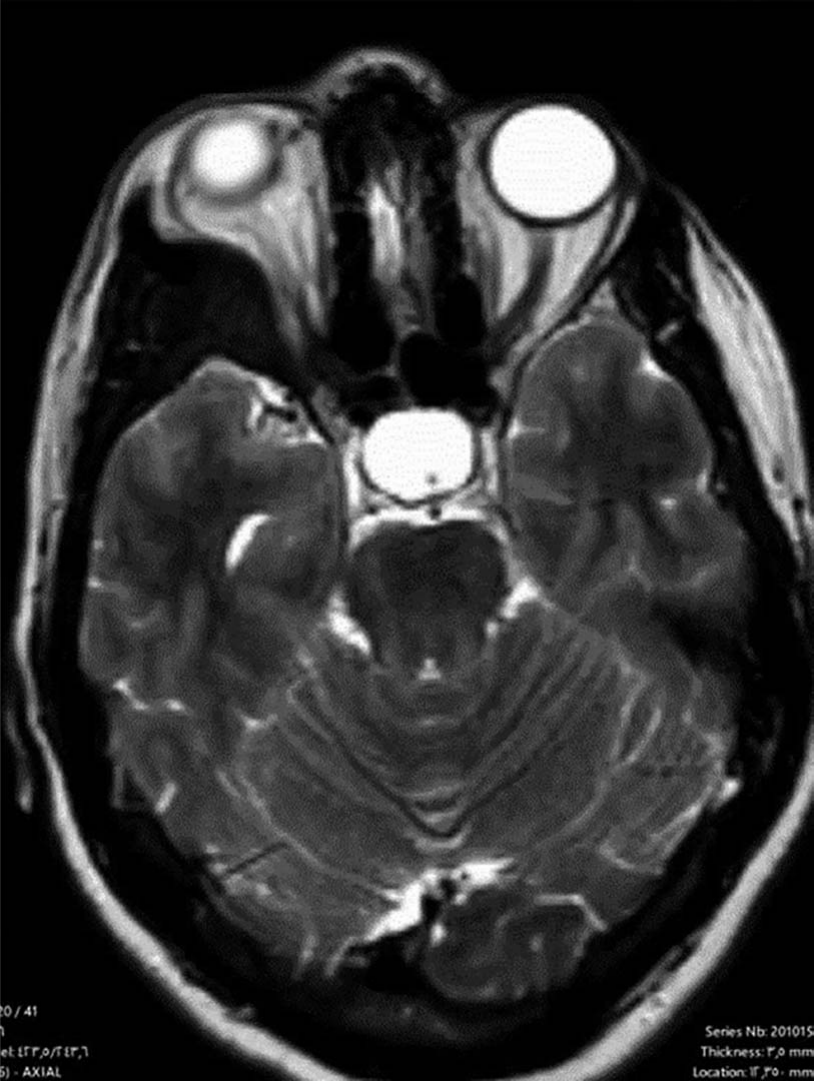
The MRI revealed a lesion with a low signal on T2-weighted in the lateral wall of the orbit compresses the rectus lateralis muscle on the right side, causing proptosis with light pressure on the anterior part of the right temporal lobe.
